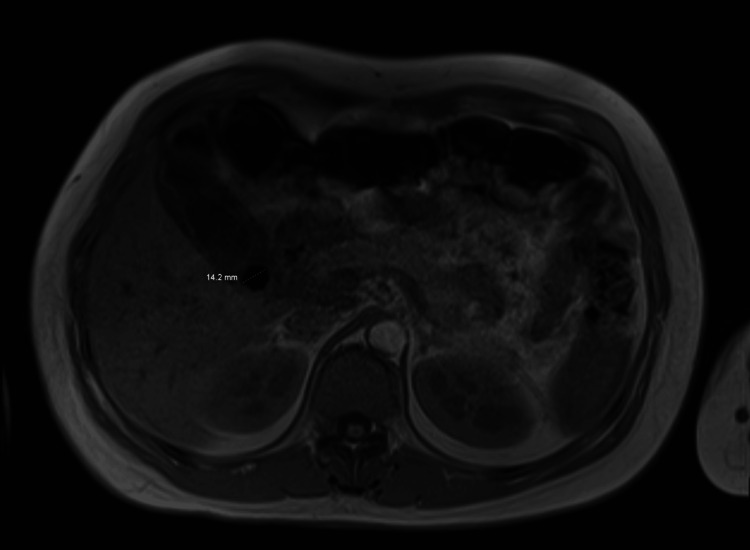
MRI abdomen Magnetic resonance imaging of the abdomen with and without contrast, demonstrating a 14.2 mm stone in the gallbladder neck
